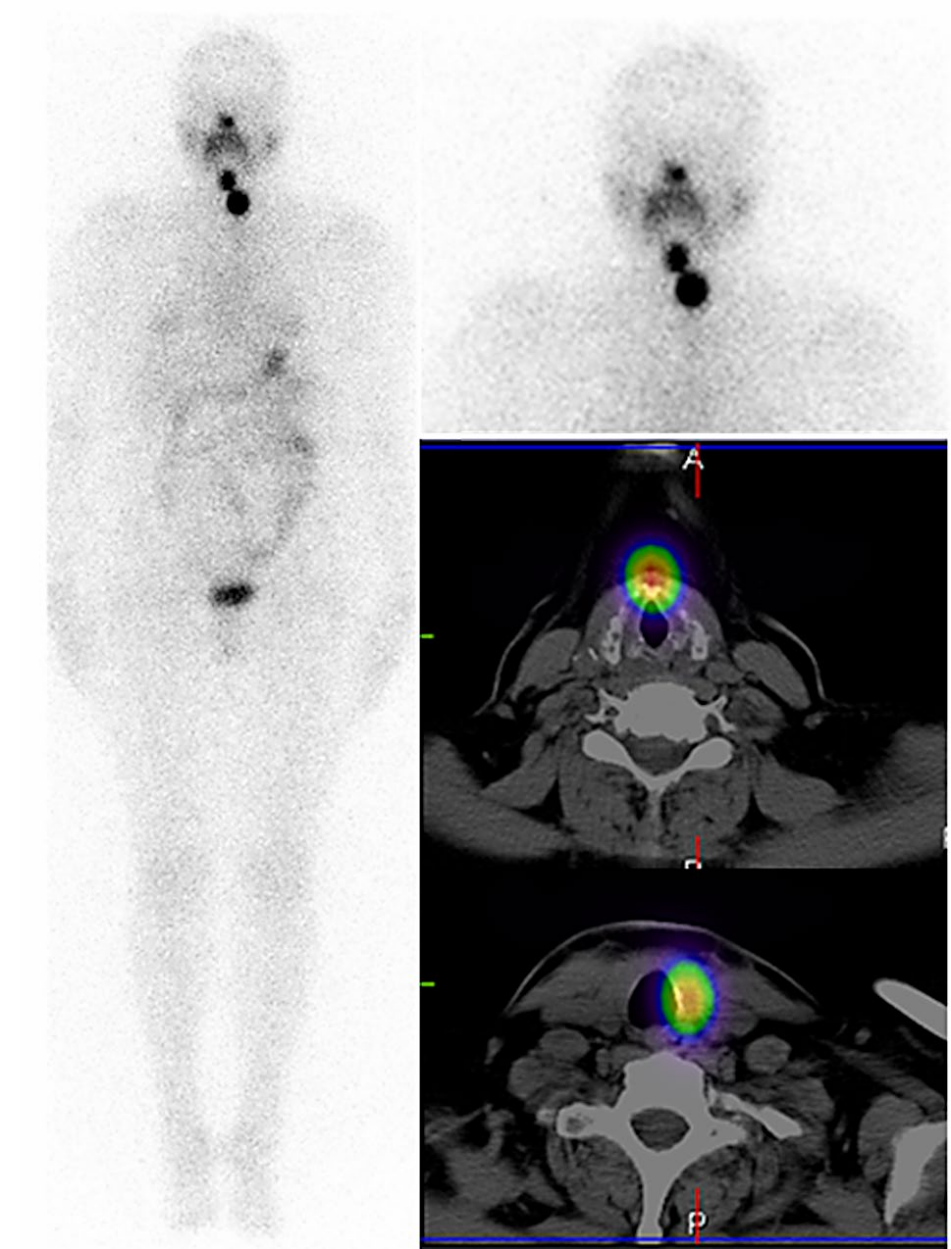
^131^I-WBS and SPECT/CT of the neck obtained 3 days after administration of 3.7 GBq (100 mCi) ^131^I in order to ablate thyroid remnants in a patient who 2 months previously had undergone complete thyroidectomy because of DTC (pT3N0M0 with vascular invasion). The images show two iodine-avid areas in the postoperative bed: one located midsagitally, at the level of the hyoid bone, most likely corresponding to the pyramidal lobe; the other one—in the left thyroid lobe bed.
